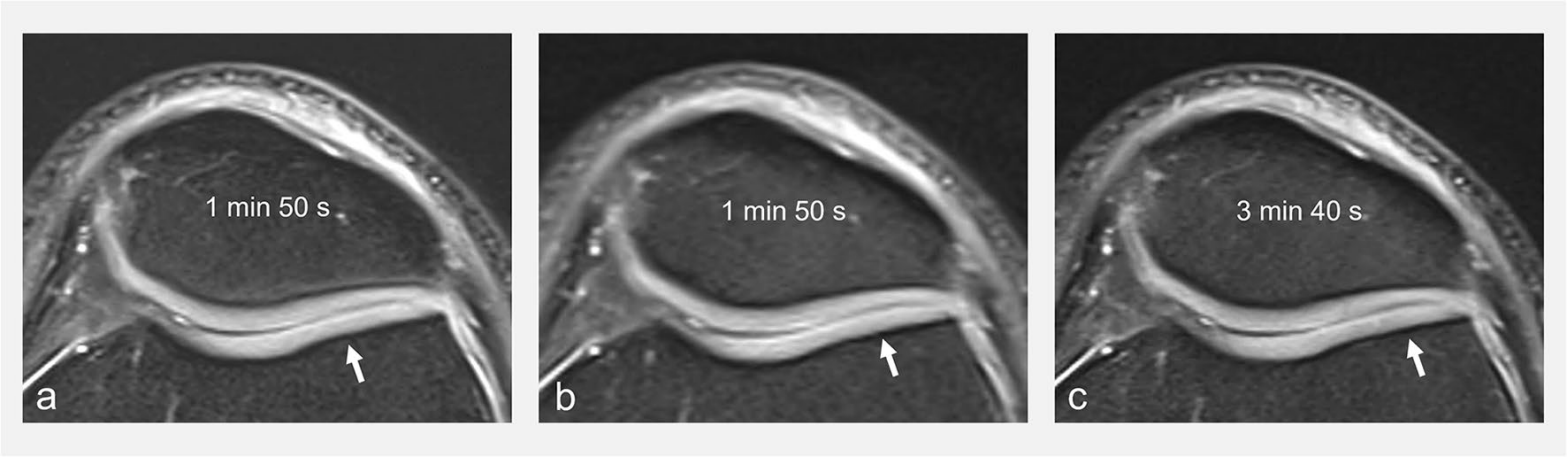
Transverse FS (CHESS) IW MR image (a), and corresponding b, c IW water Dixon MR images with different acquisition times (b, c) obtained on a 3T MRI unit. All other parameters kept equal, Dixon sequences are longer to acquire than CHESS fat-suppressed sequences (compare a and c). Note the better definition of the articular cartilage surface in a and c compared to b (arrows), due to higher spatial resolution
